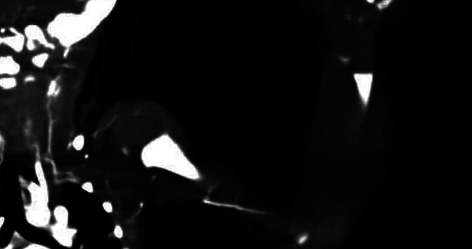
CTA shows a contrast filling defect in the left subclavian artery, starting about 2 cm from the origin of the aortic arch as a result of left subclavian artery thrombosis.
